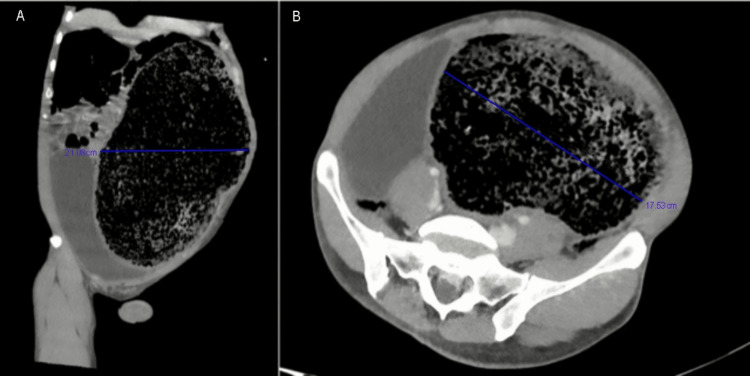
CT abdomen and pelvis with IV contrast depicting massive fecaloma. Image A highlights a fecaloma measuring 21.08 cm anterior to posterior. Image B demonstrates a transverse fecaloma measurement of 17.53 cm.
